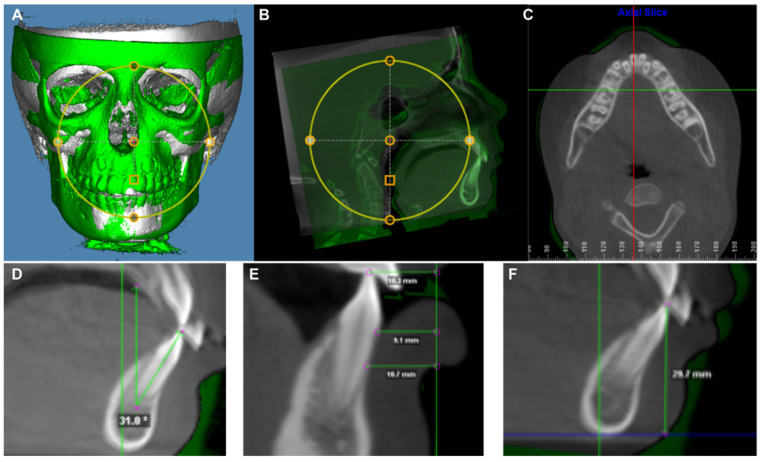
The demography of CBCT image analysis utilized in current study. (A) A screenshot of the superimposition of T1 and T2 CBCT images in a 3D reconstructed view. Th T1 CBCT image is represented in white and the T2 CBCT image is presented in green. (B) A screenshot of the superimposition of T1 and T2 CBCT images in midline sagittal slice view. The superimposition was performed based on the mandibular structure. The T1 CBCT image is represented in white and the T2 CBCT image is presented in green. (C) The axial slice was used to find the sagittal slice (red line) that was a best fit through the center of the mandibular central incisors. (D) Mandibular central incisor inclination measurement on the sagittal slice: Best fit line from tip of incisor through root apex measured to true vertical. (E) Mandibular central incisor protrusion measurement on the sagittal slice: The buccal surface of the tooth to true vertical line established at 10 mm ahead of the T1 symphysis (green vertical line). (F) Mandibular central incisor vertical position measurement on the sagittal slice: Tip of incisor to true horizontal line established at the T1 inferior border of symphysis (blue line).
